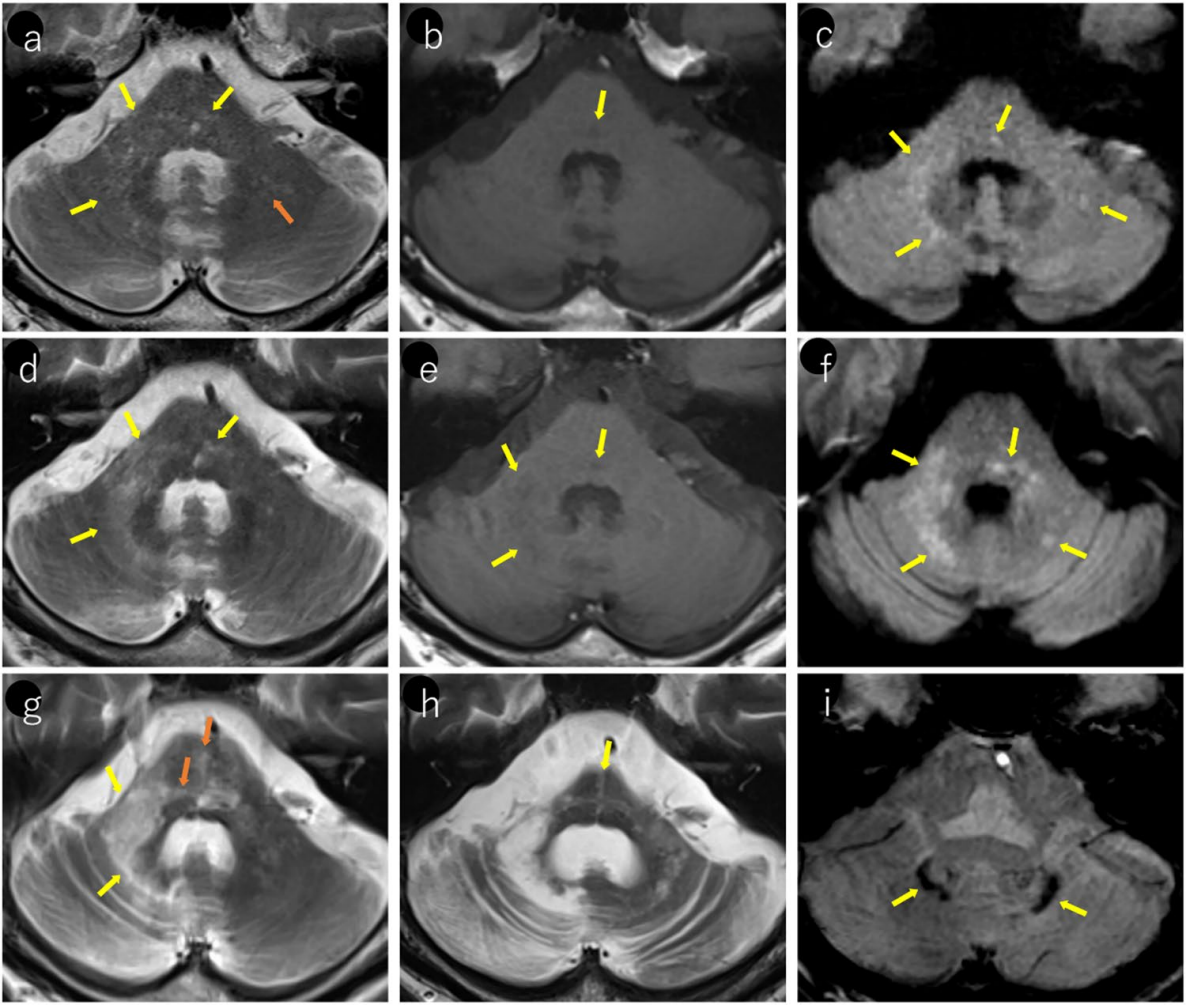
Man in his 60 s with no significant medical history presented with progressive difficulty in using chopsticks with his right hand, which began 3 months prior to presentation. Two months prior, he developed dysgraphia, followed by gradual onset of dysarthria. Initial MRI findings (a–c): a T2-weighted image showing a punctate pattern extending from the right dentate nucleus to the right middle cerebellar peduncle and pons (yellow arrows). Subtle lesions are also noted around the left dentate nucleus (orange arrow), showing an asymmetric distribution. b Some lesions appear mildly hypointense on T1-weighted images (yellow arrow). c Lesions show subtle hyperintensities on diffusion-weighted imaging (yellow arrows). One-month follow-up MRI (d–f): d lesions involving the right dentate nucleus, right middle cerebellar peduncle, and pons have expanded and demonstrated a milky way appearance (yellow arrows). e T1-weighted image showing a slight progression of hypointensity (yellow arrows). f Lesions become more conspicuous with enhanced hyperintensity on diffusion-weighted imaging (yellow arrows). JCV was detected in cerebrospinal fluid examination and PML was diagnosed. Three-month follow-up MRI (g): g T2-weighted imaging clearly demonstrates the “shrimp sign” (yellow arrows) with right cerebellar hemisphere atrophy. The pons shows a cross sign with lesion progression along the transverse pontine fibers (orange arrows). One-and-a-half-year follow-up MRI (h–i): h marked cerebellar atrophy predominantly on the right side. The pons showed atrophy with a persistent cross-sign (yellow arrow). i SWI demonstrating hypointensity in the dentate nucleus adjacent to the lesions (yellow arrows)
